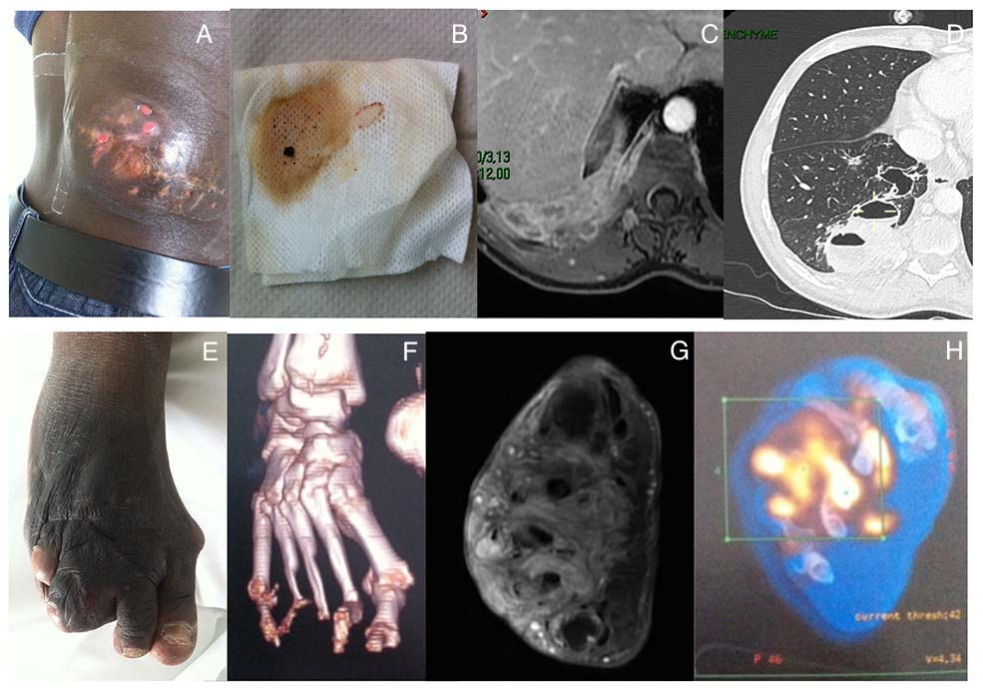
Organ involvement in patients 4 and 5. Lumbar tumefaction with draining sinuses (A). Black grains drainage (B). Paraspinal abdominal eumycetoma extension on abdomen MRI LAVA sequence (C) and lung extension on lung CT scanner (D) in patient 5. Clinical (E) second and third metatarsal destruction on 3D reconstruction CT (F) of foot. Foot mycetoma aspect on Axial T1 Fat Sat Gadolinium MRI (G) and PET/CT (H) in patient 4.
